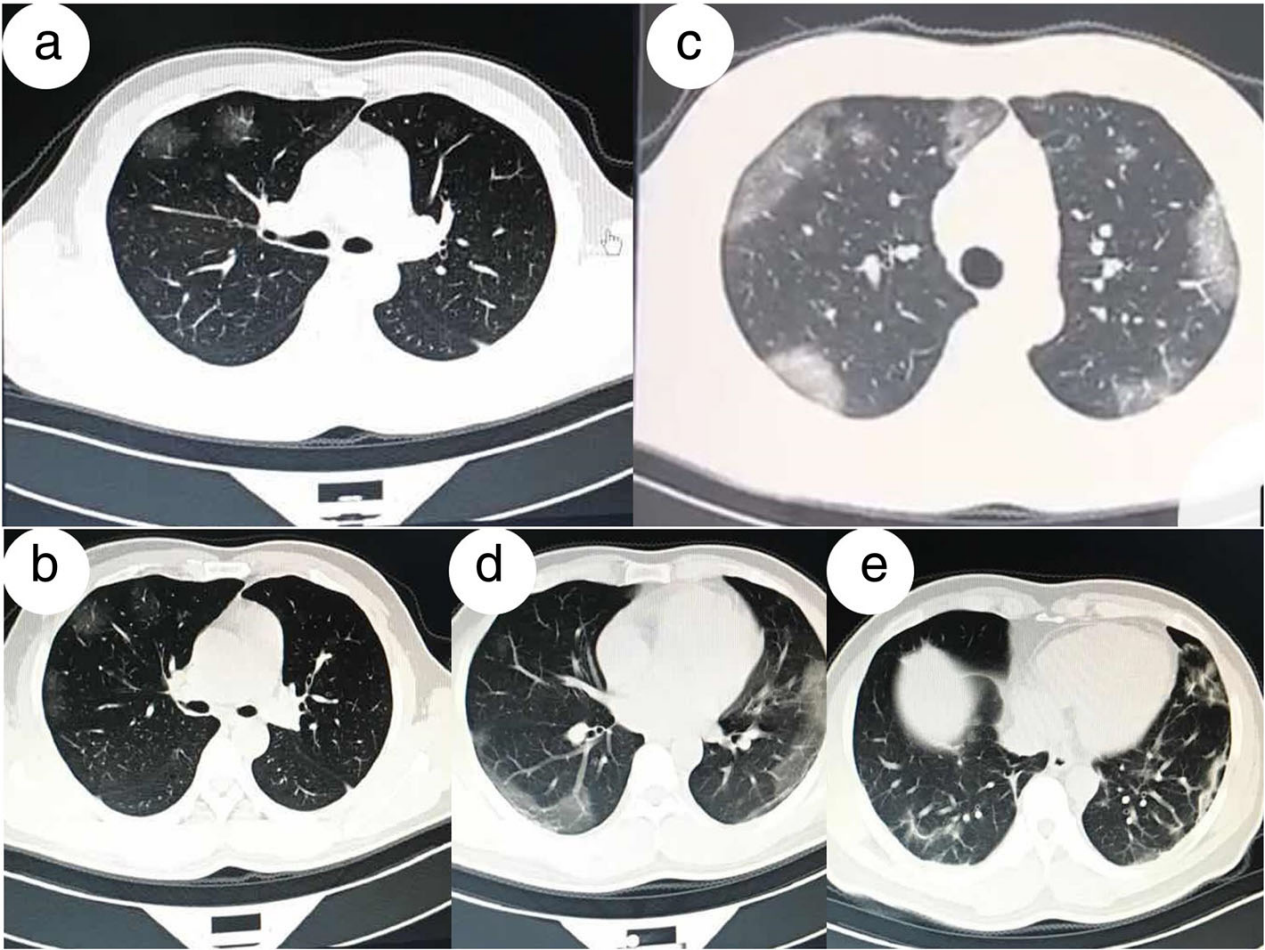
Chest imaging of the patient. a and b Chest CT scans of the lung on 30 January and 31 January 2020 (3–4 days after the symptom onset) revealed slight pulmonary infiltration. c and d CT scans taken on 3 February 2020 (8 days after the symptom onset) showed obvious pulmonary infiltrates. e CT scans taken on 10 February 2020 (15 days after the symptom onset). HRCT, High-resolution computed tomography
